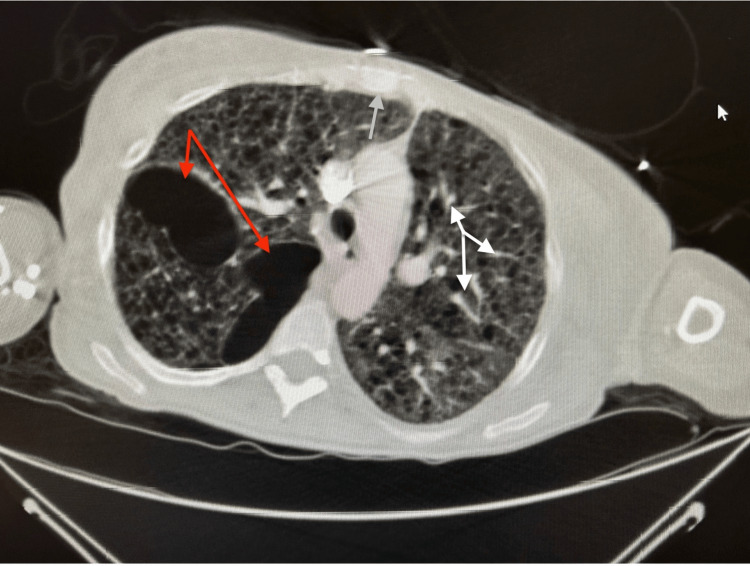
Chest CT reveals LAM, pulmonary infiltrates, and thoracic wall soft tissue edema CT: Computed tomography; LAM: Lymphangioleiomyomatosis; Red arrows: LAM; White arrows: Pulmonary infiltrates; Grey arrow: Thoracic wall soft tissue edema
